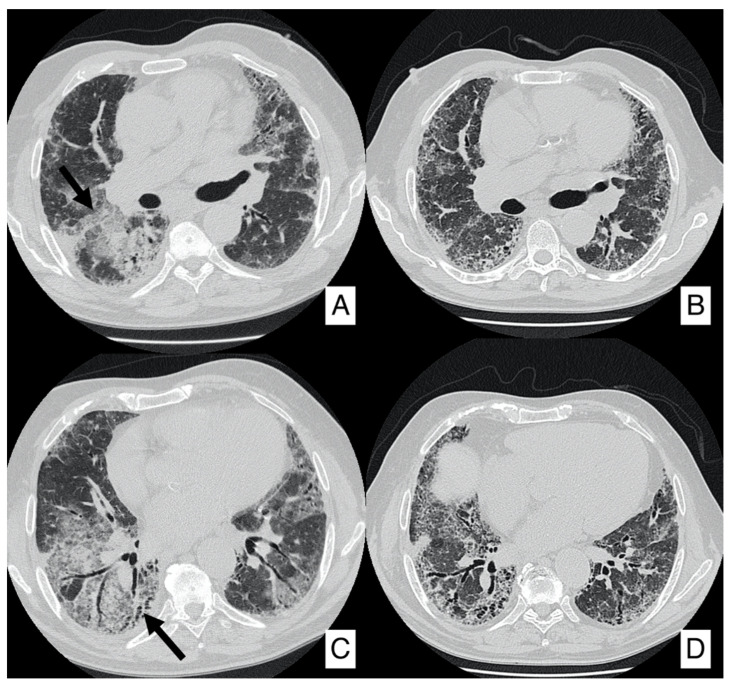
Same IPF patient as Figure 8: comparison between CT acquired during exacerbation (A,C) and follow-up scan (B,D), the latter performed 4 months later. Images below the carena (A,B) demonstrate resolution of ground-glass opacification of the right lung (apical segment of the lower lobe, black arrow); similarly, images acquired at basal levels clearly show that ground-glass attenuation of the lower lobes (black arrow for the right lower lobe) decreased at the follow-up evaluation.
